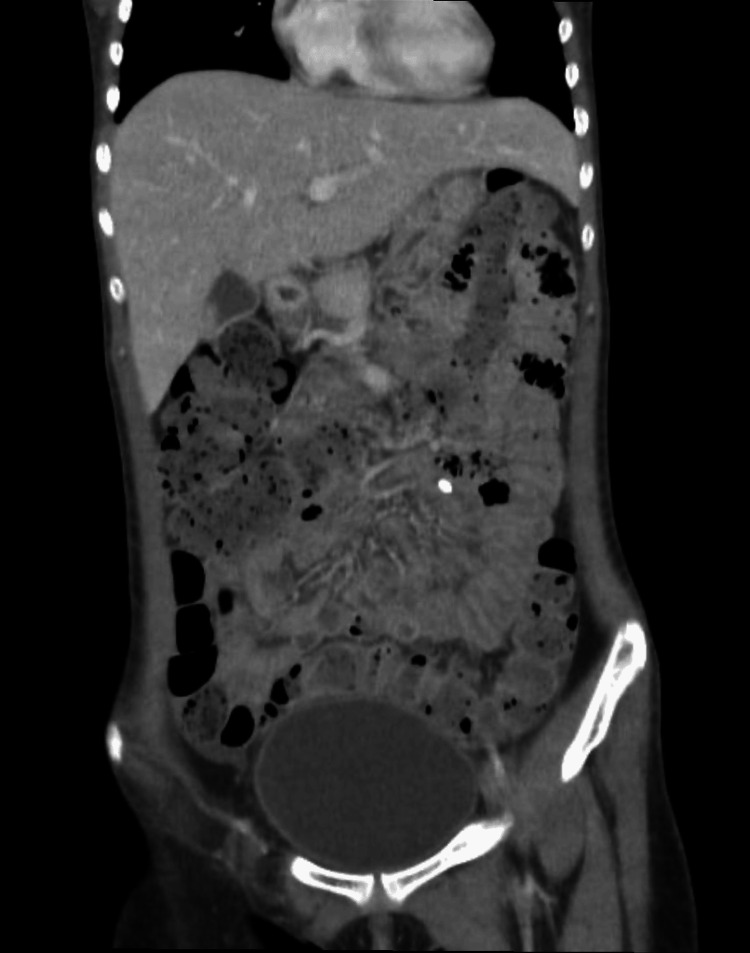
Baseline contrast-enhanced abdominal CT at initial presentation (Day 0) The stomach is of normal caliber, without distension, and no free intraperitoneal fluid is present; incidental hyperdense gallbladder content consistent with microlithiasis was also noted. No arrow is shown, as this baseline study demonstrates no focal abnormality. CT, computed tomography.
